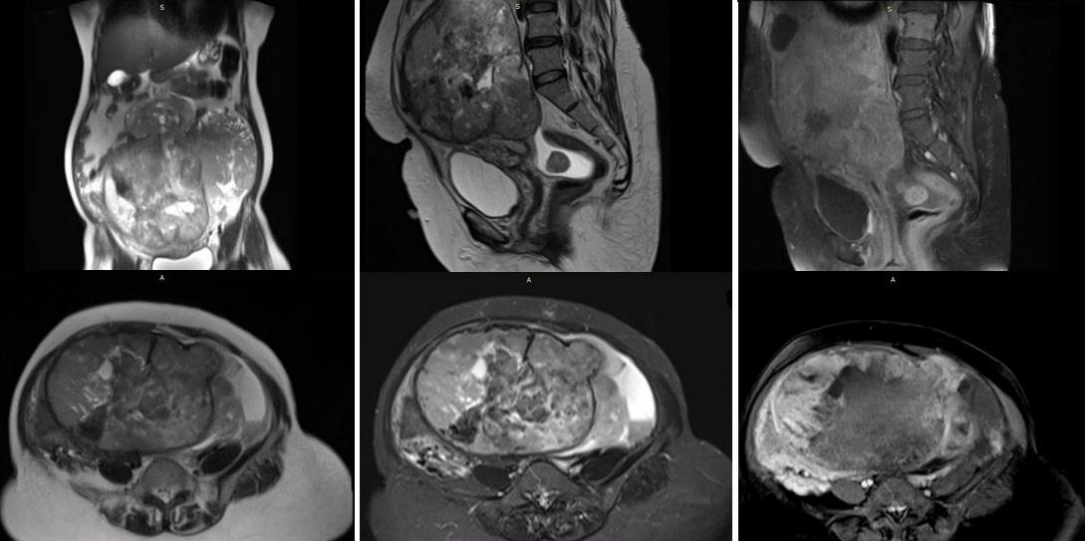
preoperative MRI pictures of the lower abdomen showing the presence of a large abdomino-pelvic tumour originating from pelvic cavity
